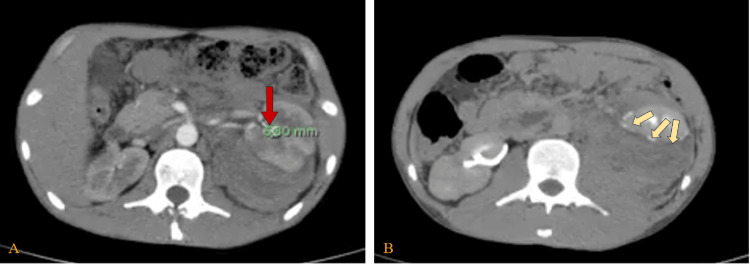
Repeat abdomen and pelvic CT with IV contrast on day 5 (axial view) (A) Arterial phase showing haematoma decreased in size slightly and increase in the size of contrast blush at the mid pole posteromedially represents an active bleeding (green mark and red arrow). (B) Delayed phase showing a rim of contrast extravasation around the left kidney (yellow arrows) with suspected cortical defect reaching its pelvic seen in the midportion and hypoenhancing area in the upper pole, suggesting urinary extravasation
